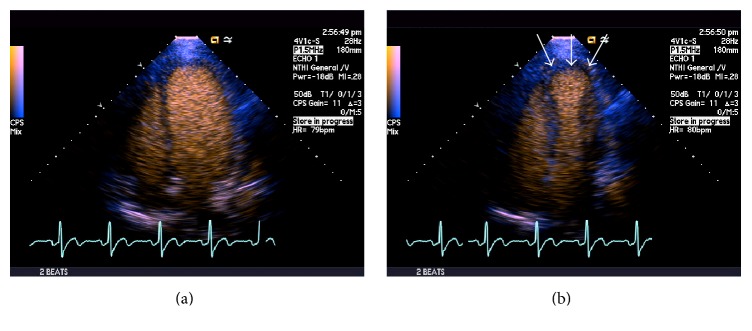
Apical four-chamber echocardiographic images with contrast enhancement at end-diastole (a) and end-systole (b) showing severe akinesis of the left ventricular apex (arrows).
